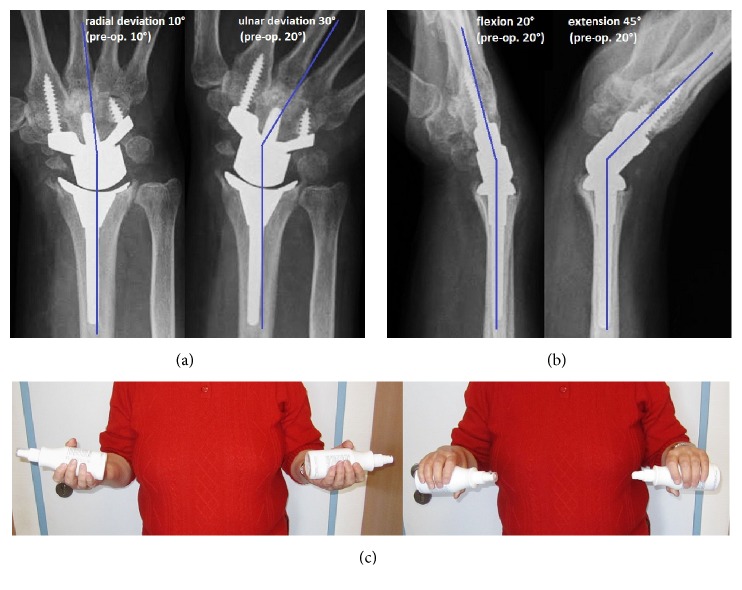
Case report (3-year follow-up): (a) PA radiographs showing no recurrence of GCT, unchanged correct alignment of TWA without any signs of loosening, and no impingement with terminal ranges of motion; (b) lateral PA radiographs with terminal ranges of motion showing no instability of TWA; (c) clinical photographs demonstrating 90° supination and 90° pronation of both forearms.
